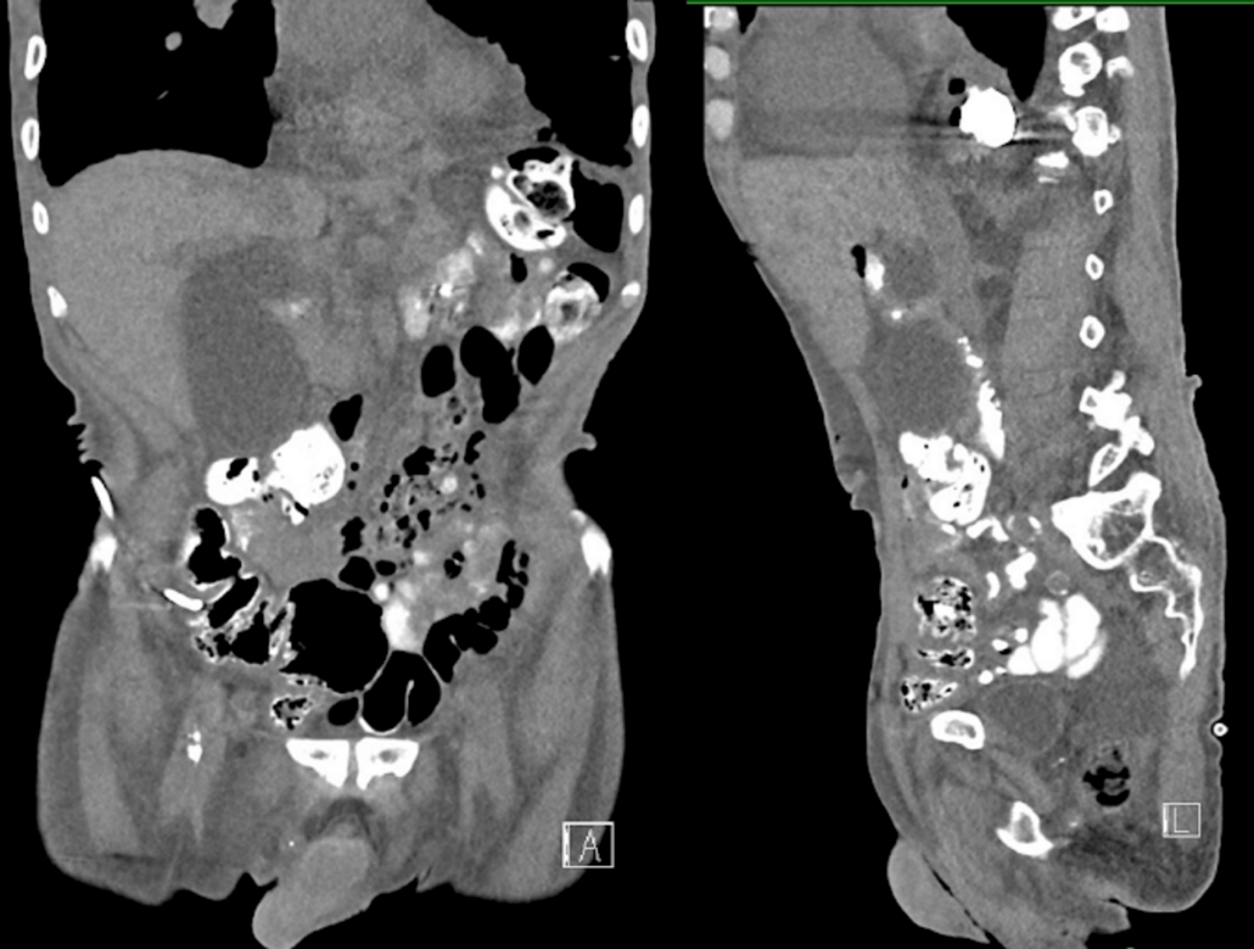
Computed tomography (without contrast) of the abdomen following drainage of the patient’s right renal cyst with coronal and sagittal views.
